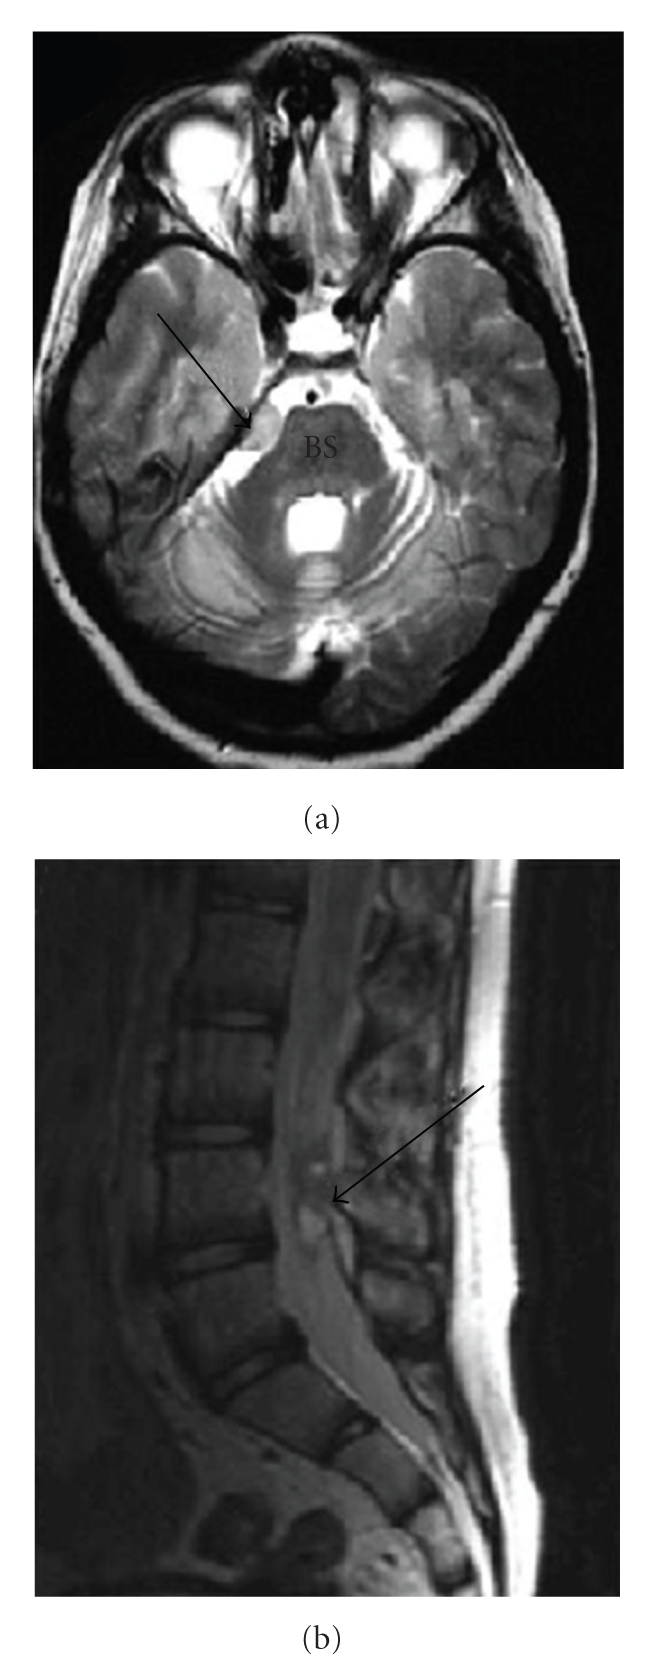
(a) Brain MRI demonstrating a lesion (arrow) based on the tentorium next to the brain stem (BS). (b) Spinal-lumbar MRI (T2) showing an intradural lesion (arrow) at the level of the L4 vertebra. Reproduced with permission.
